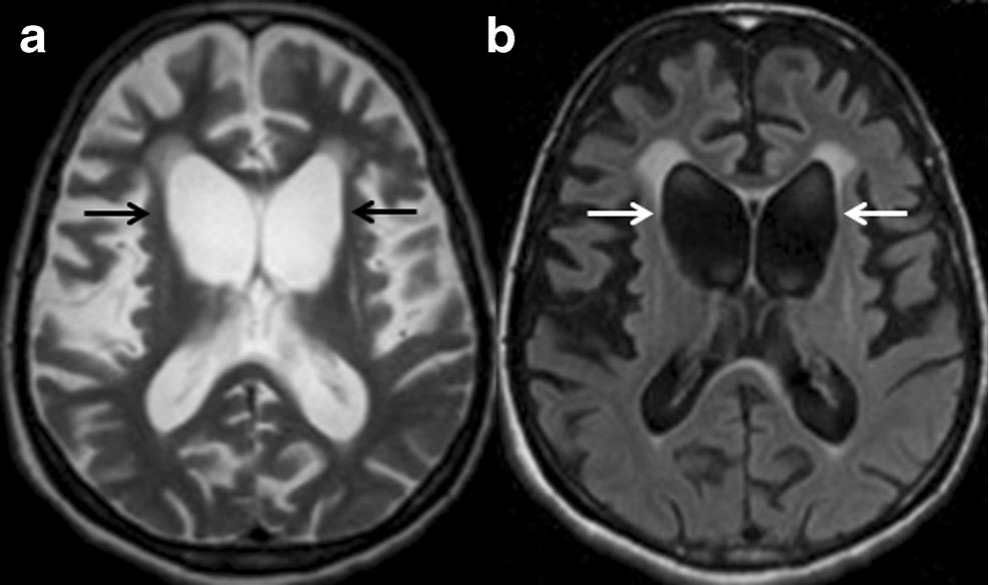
Huntington’s disease. A 62-year-old male presented with abnormal choreiform movement of the limbs, psychiatric manifestations and dementia. a axial T2W image at the level of lateral ventricles is showing significant atrophy of bilateral caudate nuclei (*black arrows*) and frontal lobes. b corresponding axial FLAIR image also demonstrates significant caudate atrophy (*white arrows*) and enlargement of frontal horns
